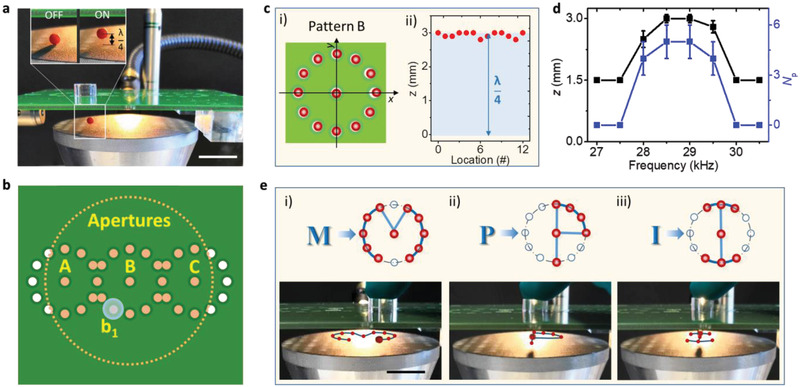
EPS particle levitation and positioning by blocking apertures at different locations. a) Levitation of an EPS particle by switching the applied ultrasound ON/OFF. b) Top view of the experimental setup, including the polymethyl methacrylate (PMMA) block b1 (transparent blue, top layer), the aperture plate (green, middle layer) fabricated with three identical aperture patterns (A, B, and C) and the ultrasound transducer (pink, bottom layer) placed below the aperture plate. The pink dashed circle shows the position for the transducer. c) Manipulation test for trapping (i) and levitating (ii) a single particle by individually blocking apertures at different locations. d) Dependence of levitation height (*z*) and capacity (*N*
